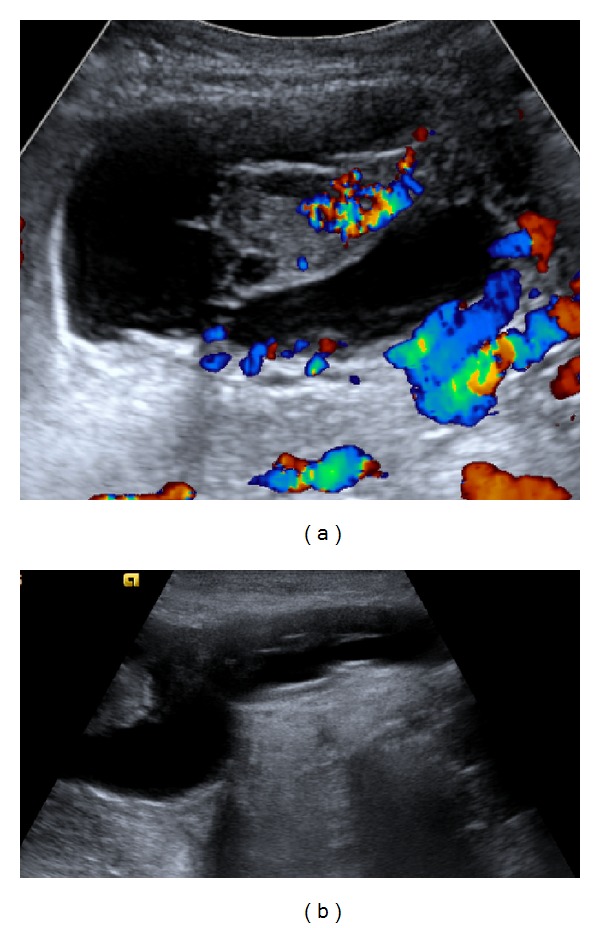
(a) Ultrasound demonstrating an avascular cystic collection in the right adnexal region, containing ovarian tissue that is connected to the peritoneum by a pedicle. (b) Ultrasound demonstrating extension of cystic mass into the right inguinal canal.
